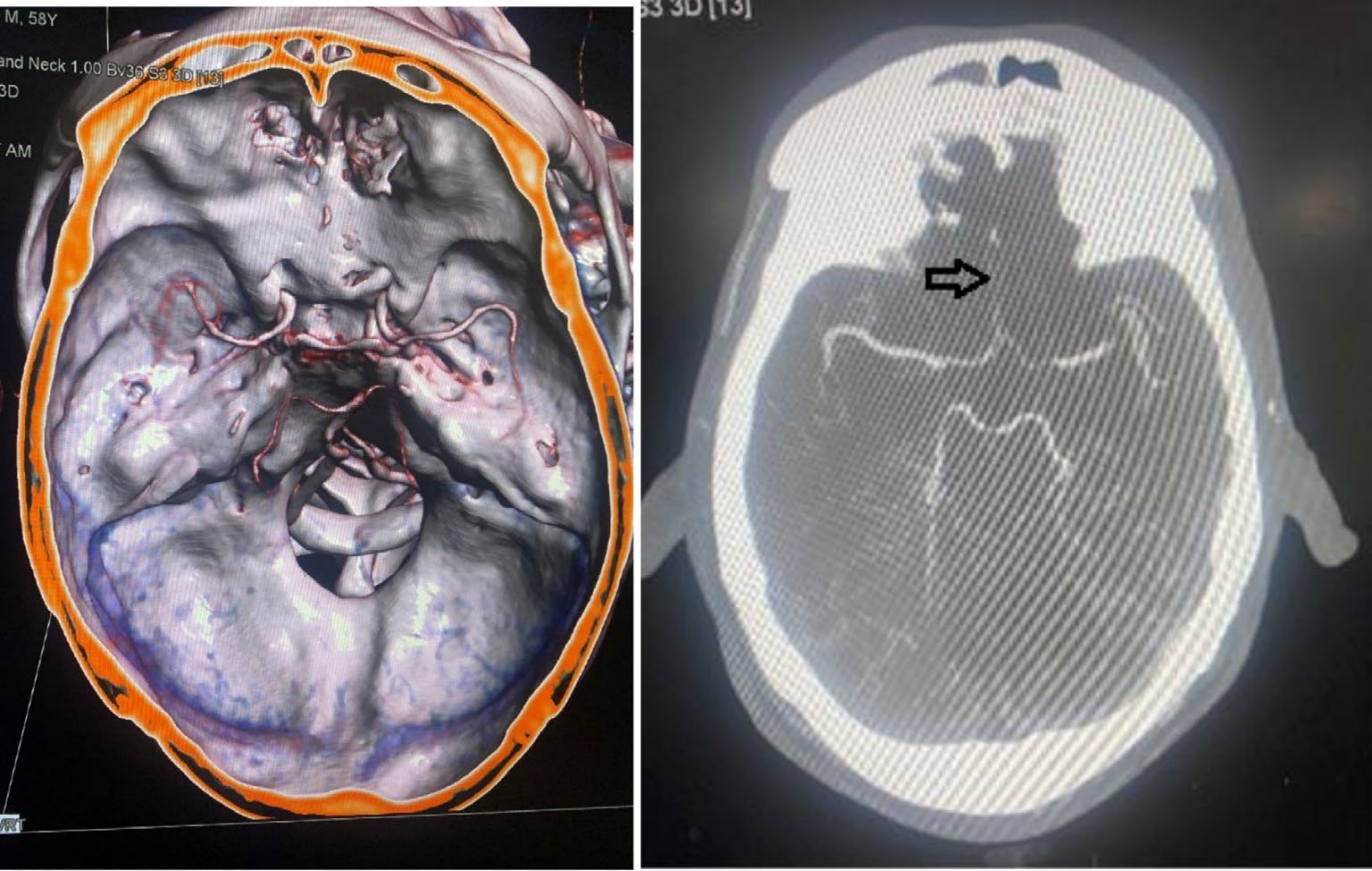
CT‐angiogram showing non‐opacification of A2 and attenuated caliber of A1 segment of anterior cerebral artery (ACA).
